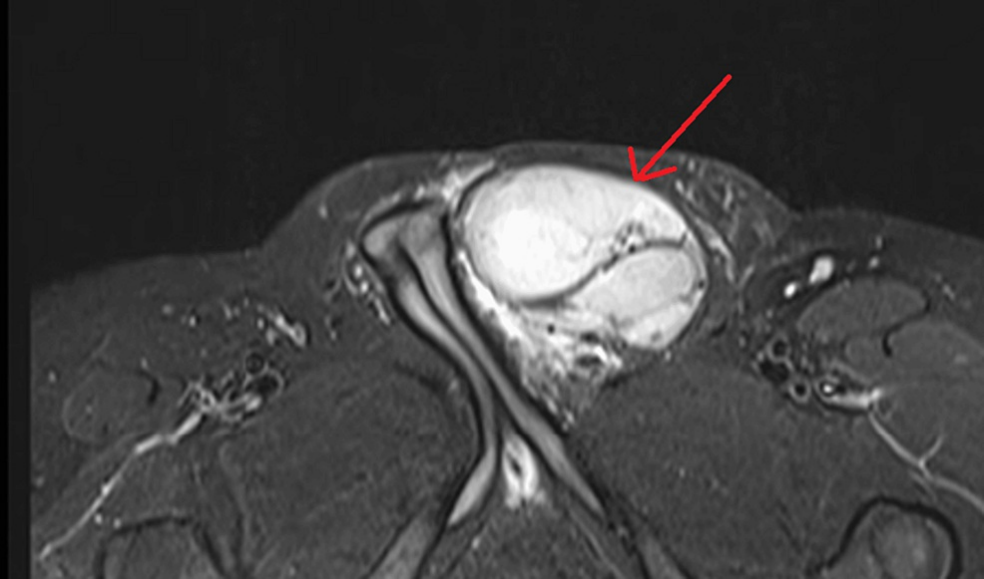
MRI T2 fat saturated axial section shows a multilobulated lesion with heterogenous signal in left para testicular region.
